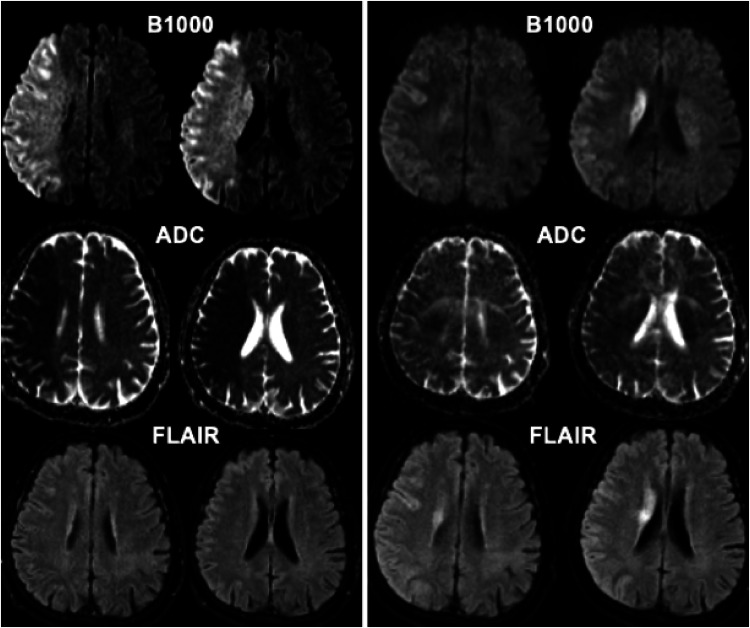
Baseline (left) and post-thrombectomy (right) MR images in a 60-year-old patient who presented with acute right MCA syndrome with M1 occlusion (not shown). Using ADC <620 volume of ischemic core on baseline imaging was calculated at 273 mL. MRI following successful reperfusion (mTICI 2b) obtained at 48 h post-thrombectomy shows significantly smaller infarct volume of 104 mL on B1000 images. ADC: apparent diffusion coefficient.
